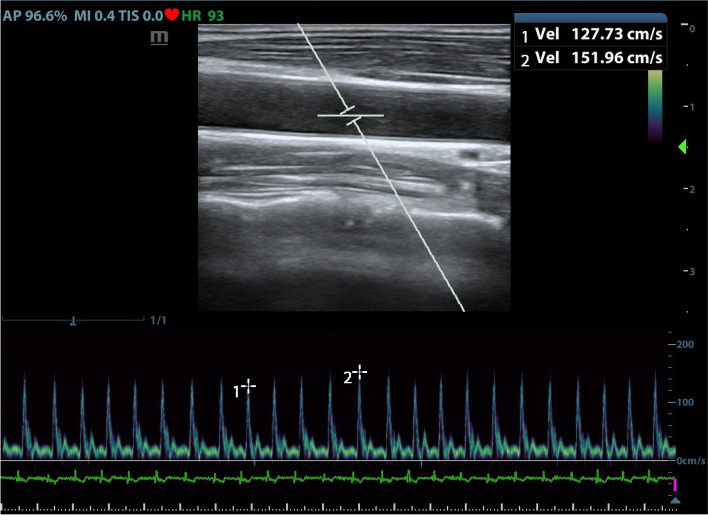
Ultrasound measurements of the carotid artery and calculation of ΔVpeak. The panel above shows a two-dimensional scan of the right common carotid artery, and the following panel shows the corresponding blood flow spectrum. “1 vel” and “2vel” represent the minimum and maximum values of peak blood flow velocity, respectively, in a single respiratory cycle
